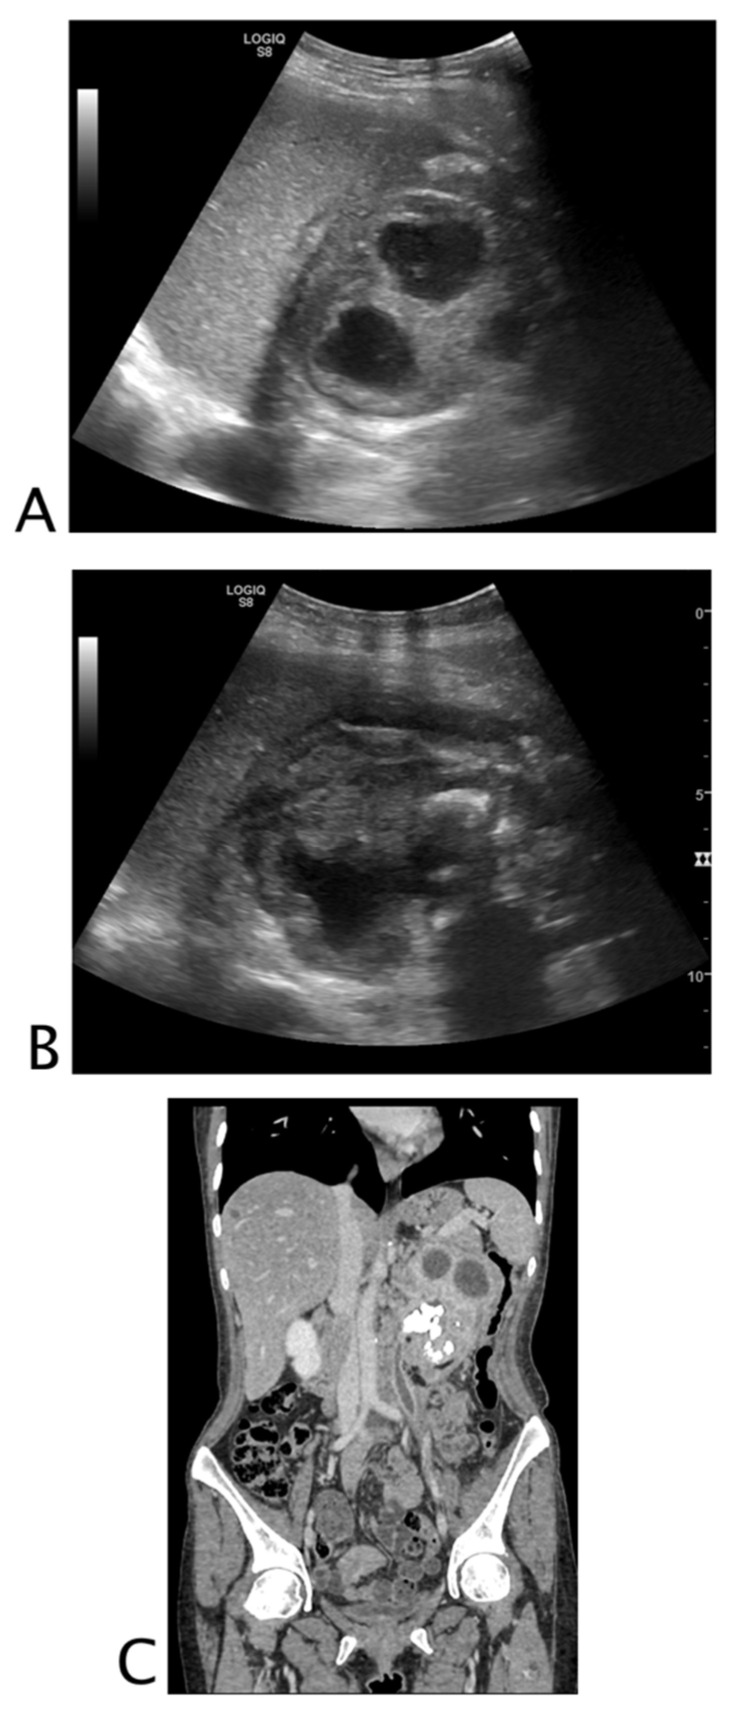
Black is too much, black is not clearly black, and black is where it should not be. A 47-year-old asthenic female patient with unintentional severe weight loss and left flank pain. At ultrasound, the left kidney was hardly recognizable; dilated upper calices with thick parietal walls and inhomogeneous urine (A) on the lower pole staghorn calculi were detected (B). Perinephric and subcapsular fluid was visualized. A suspected ultrasound diagnosis of complicated pyonephrosis was formulated and CT was performed in order to stage the pathology. (C) Final CT diagnosis: stage III xanthogranulomatous pyelonephritis with iliopsoas and lumbar muscle invasion.
